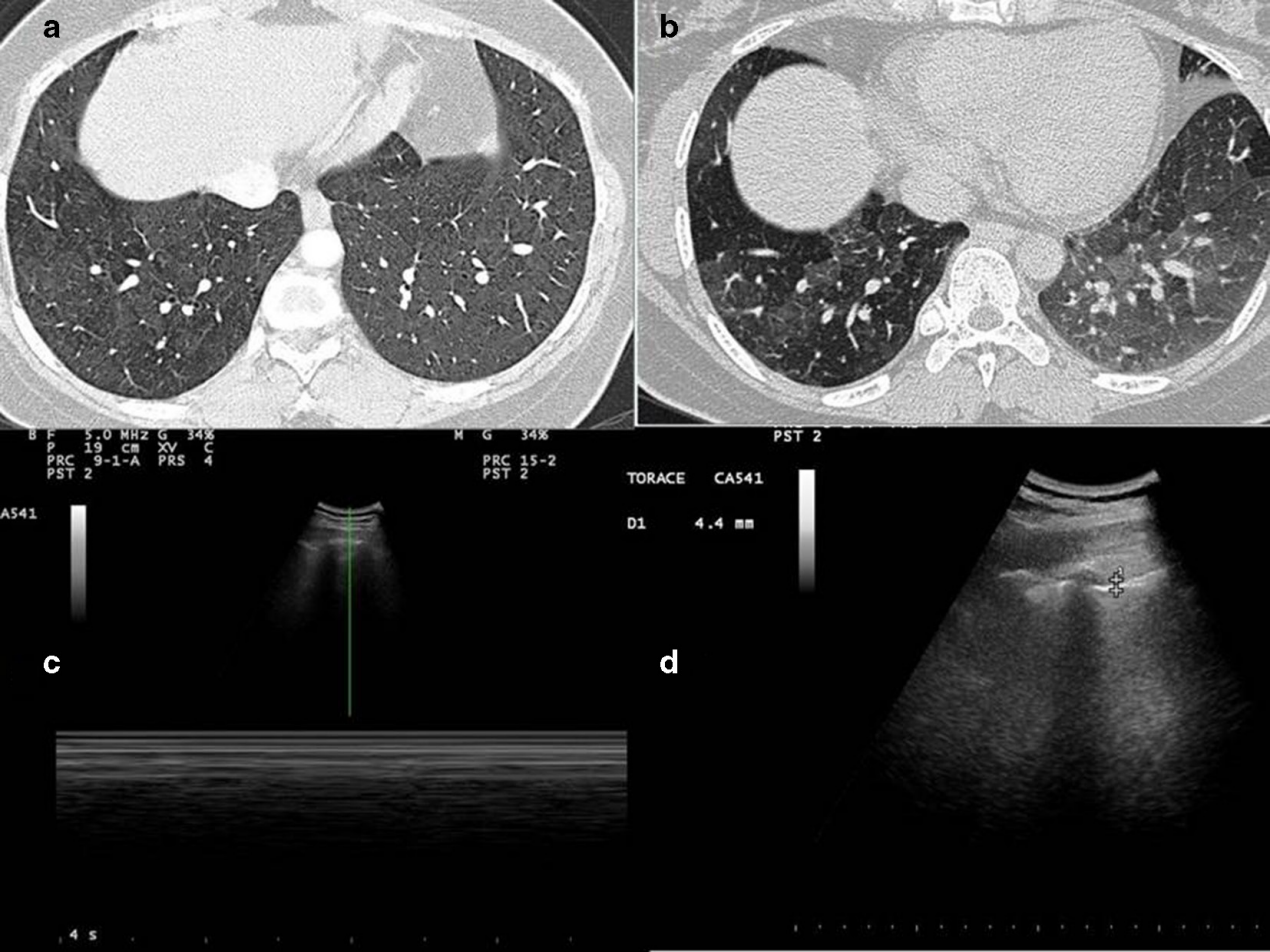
(A, B) HRCT scans show minimal thickening of the bronchial walls and a slightly inhomogeneous appearance of the lung parenchyma (a mild picture of “mosaic oligohemia”). (C) Lack of gliding sign is confirmed by Barcode sign in M-mode with convex probe (5 MHz). (D) Ultrasound imaging with convex probe (5 MHz) shows an irregular thickening of the hyperechoic pleural line (3.1–4.4 mm). HRCT, high resolution CT.
